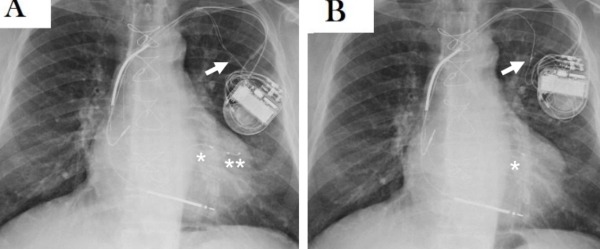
A) Chest PA X-ray showing the tip (*) of the unipolar CS lead fracture (arrow) near the tip of the bipolar CS lead (); B) Chest PA X-ray showing the unipolar CS lead terminal repaired (arrow).
